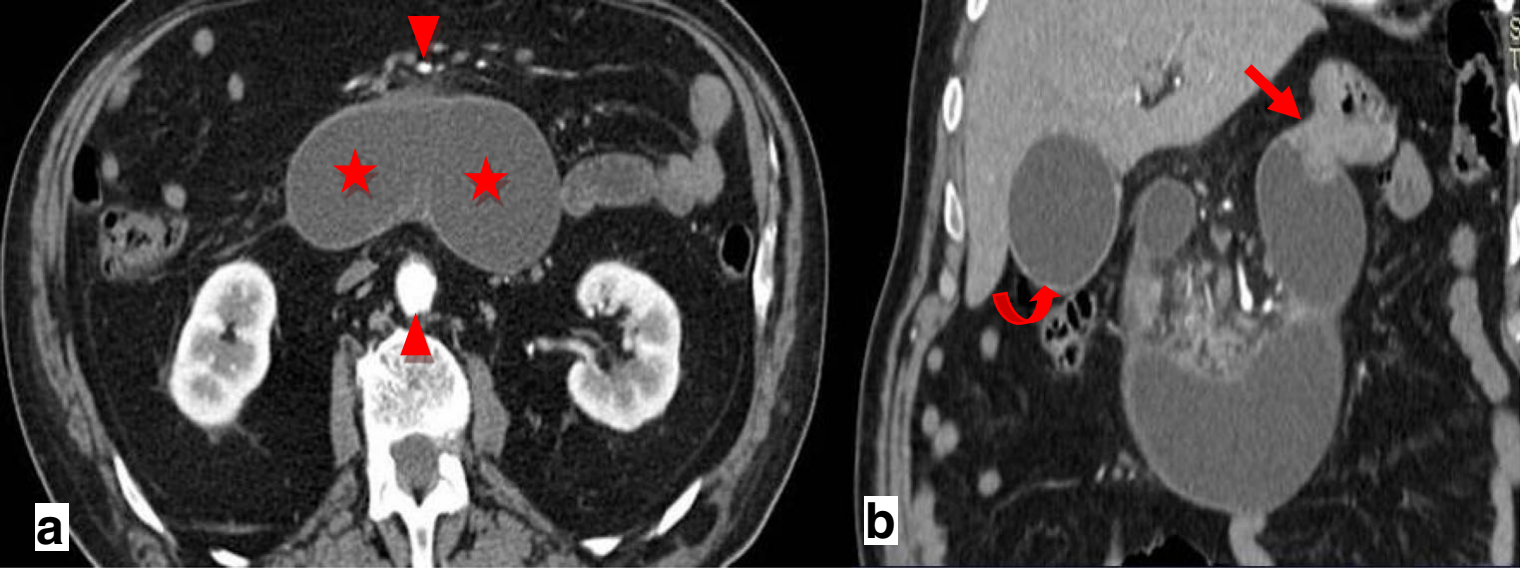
a, b Abdominal CT of the same patient as in Fig. 13. A fluid-filled tubular structure (*stars in a*) crossing the midline between the aorta and the superior mesenteric vessels (*arrowheads in a*) is seen, with associated gallbladder distension (curved arrow *in b*)–afferent-loop syndrome caused by tumor recurrence, depicted as an irregular enhancing mass at the anastomosis site (*arrow in b*)
